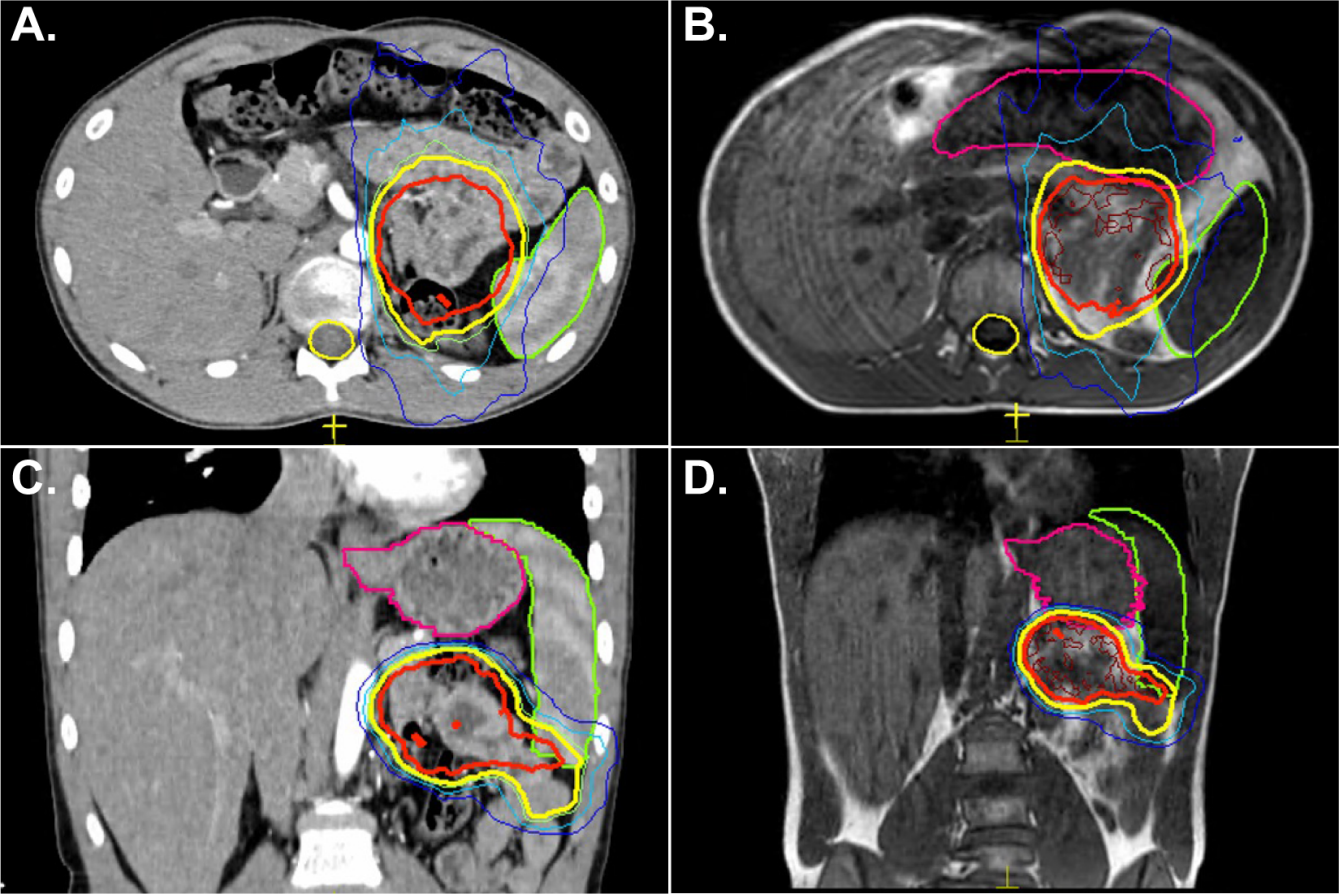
A 16-year-old male with history of kidney transplant and lymphoma presented with tarry stools and was found to have lymphoma recurrence in the jejunum as the only sites of disease. He received chemotherapy followed by radiation treatment to 45 Gy in 25 fractions. Panel (A) shows the radiation treatment plan on CT where the structure in green represents the spleen, the yellow line represents the 39 Gy isodose line, and the red line represents the 45 Gy isodose line. Panel (C) shows the initial treatment plan and the relationship to the stomach, outlined in pink. Panels (B, D) are a representative example of change in stomach shape while the patient underwent treatment. Without adapt-to-shape capability, the stomach would have received a higher dose than desired.
